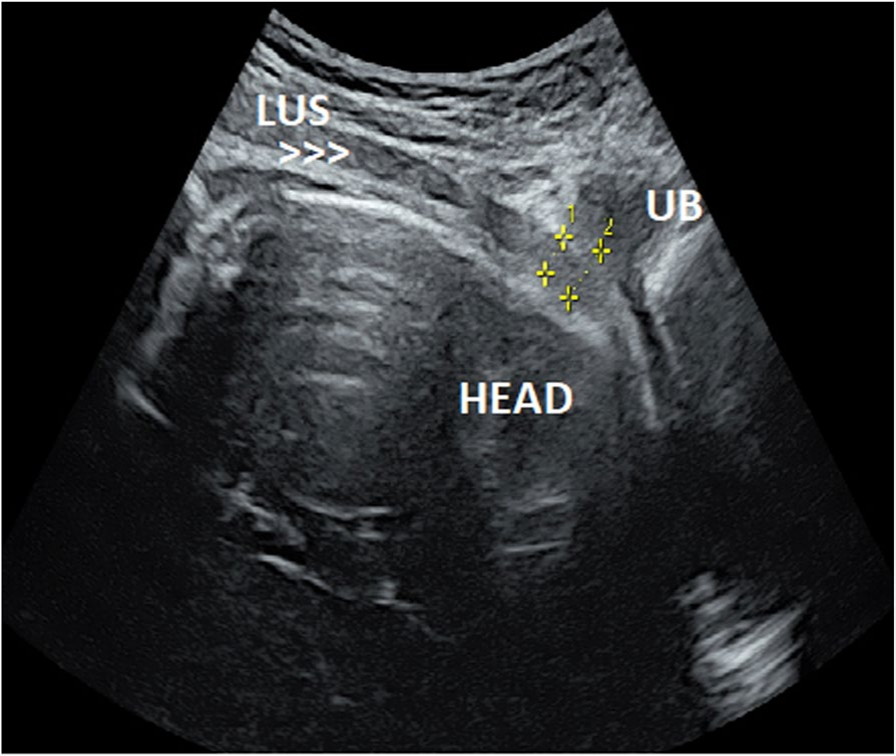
Trans abdominal ultrasound during first stage of labor with partial empty bladder, measuring the Lower Uterine Segment (LUS) with posterior UB wall, which shows a decreased thickness of the LUS (1.5 mm). M: myometrium, F: fetus head
